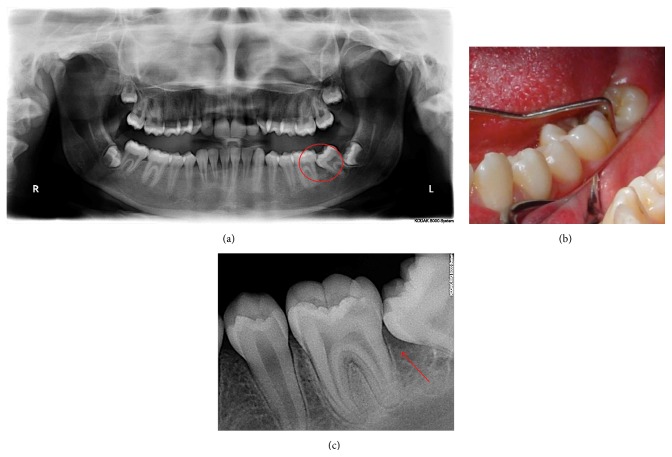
(a) Radiographic view of baseline (red circle showing defect side), (b) clinical view of defect side with periodontal probe, and (c) radiographic view of defect side (red arrow showing bone level).
